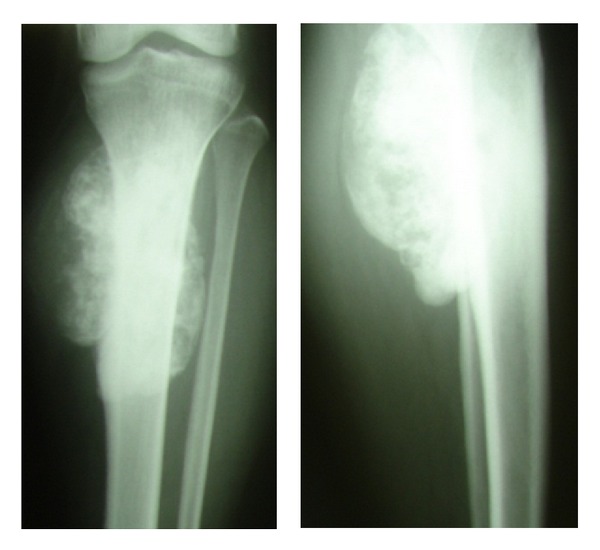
Anterior-posterior and lateral radiographs of the proximal tibia showing a sclerotic lesion suggestive of parosteal osteosarcoma.
